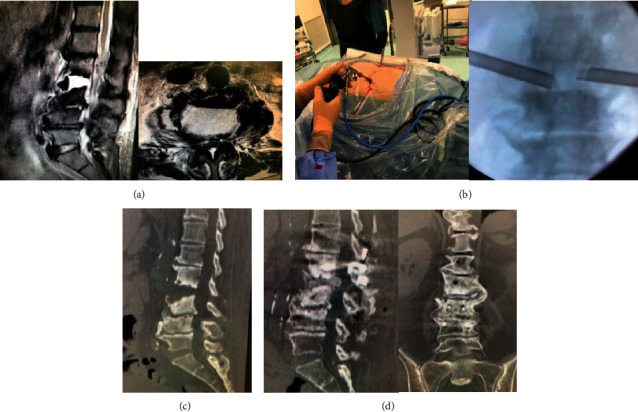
A 71-year-old female was referred because she had severe low back pain after treatment at the Department of Internal Medicine due to urinary tract infection. After spinal endoscopic surgery, low back pain persisted and the patient found difficulty in getting out of the bed; therefore, anterior-posterior lumbar fusion was performed. After the operation, the low back pain improved. (a) Sagittal and axial T2-weighted MRI; an abscess that had formed an air fluid level is identified at the L2/L3 level. (b) Intraoperative view and fluoroscopic image; two portals are inserted on both sides of the intervertebral space, and debridement and irrigation were performed endoscopically. (c) Post-FED sagittal CT scan image showing progress of bone destruction. (d) Post-open surgery sagittal and coronal CT scan view; bone fusion at L2/L3 level was observed 3 months after the minimally invasive open surgery.
